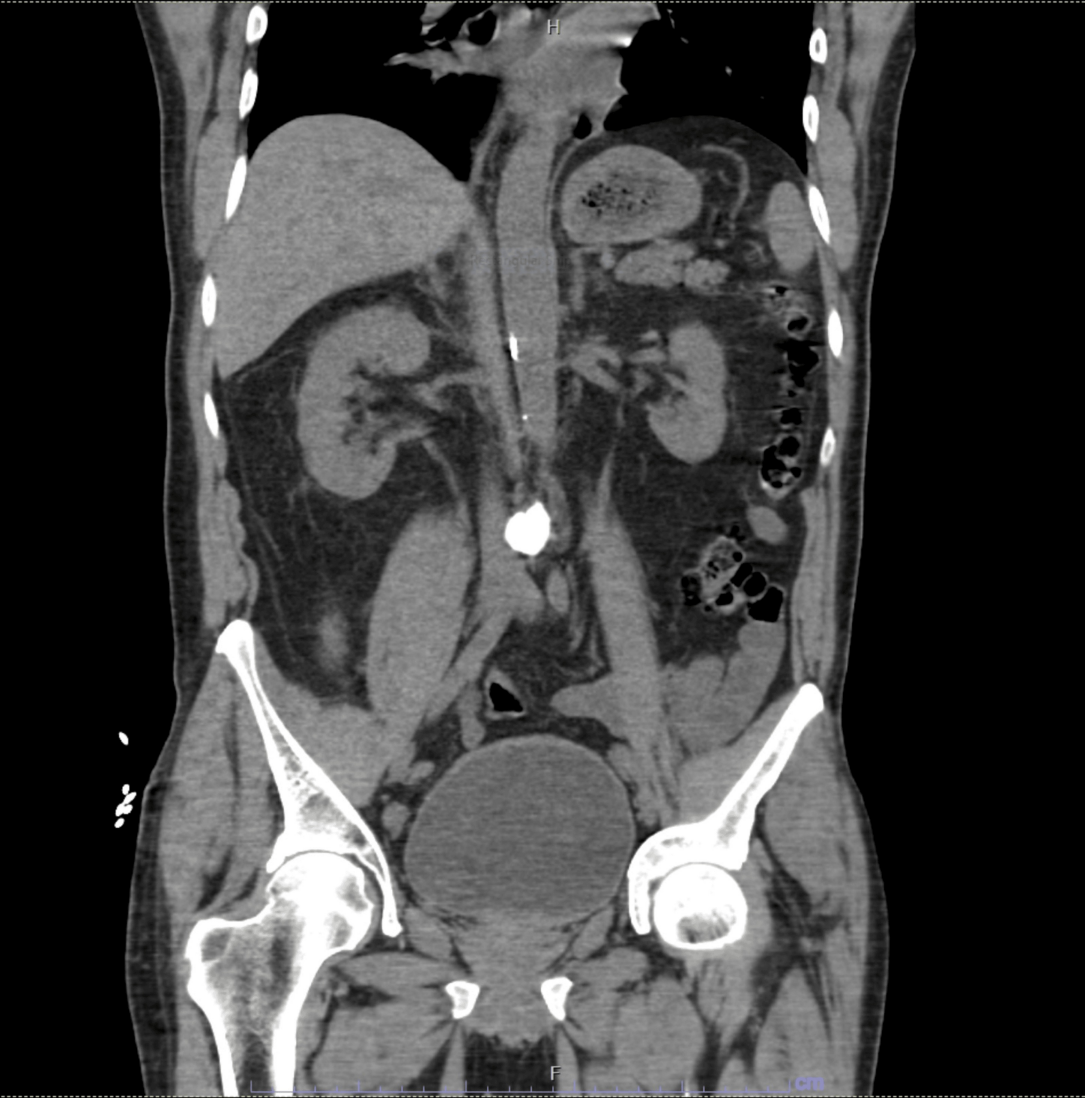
Coronal view of CT abdomen pelvis without IV contrast
